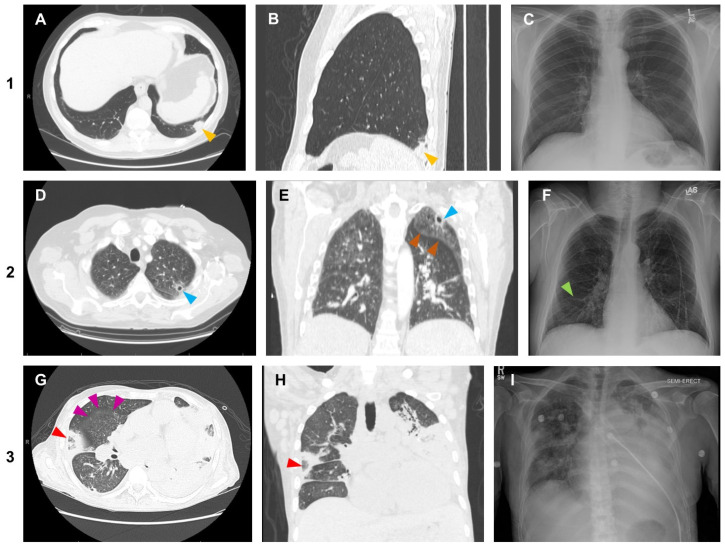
Representative radiologic images from patients with (1) an IPM-suspicious CCT but normal CXR, (2) IPM-suspicious CCT with abnormal but discordant CXR, and (3) matching IPM-suspicious lesions on CCT and CXR. Patient 1: (A,B) CCTs showing a 2 cm solid nodule in the left lower lobe (yellow arrowhead). Other CCT images not included in this figure revealed ground glass opacities with nodules in the right lower lobe, indicative of multifocal infection. (C) Largely normal CXR without signs of pneumonia. Patient 2: (D,E) CCTs showing bilateral ill-defined ground glass opacities (brown arrowheads) and nodules predominating in the upper lobes, one of which is cavitating (blue arrowheads). (F) CXR not revealing the lesions seen on CCT but showing linear opacities in the right middle lobe (green arrowhead) and slightly increased opacity in the right apex. Patient 3: (G,H) CCT showing bilateral pneumonia with multifocal consolidation and opacities (e.g., in the area highlighted with purple arrowheads) and nodules/consolidations with reverse halo morphology (red arrowheads). (I) CXR showing bilateral airspace disease with numerous opacities and nodules that are consistent with the CCT findings. Abbreviations: CCT = chest computed tomography; CXR = chest X-ray; IPM = invasive pulmonary mucormycosis.
